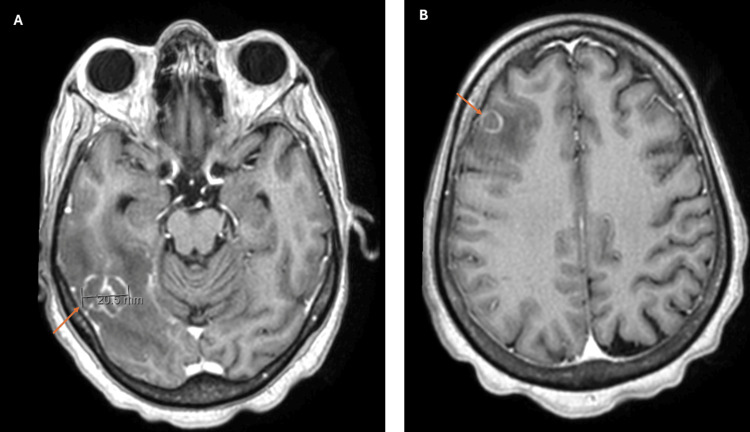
Brain MRI scan Two axial T1-weighted images (A and B) of brain MRI showing multiple ring-enhancing lesions (arrows). The frontal lesion has sub-centimetric diameter (B), and the lesion in the inferior and posterior right temporal region measures approximately 2 cm (A).
